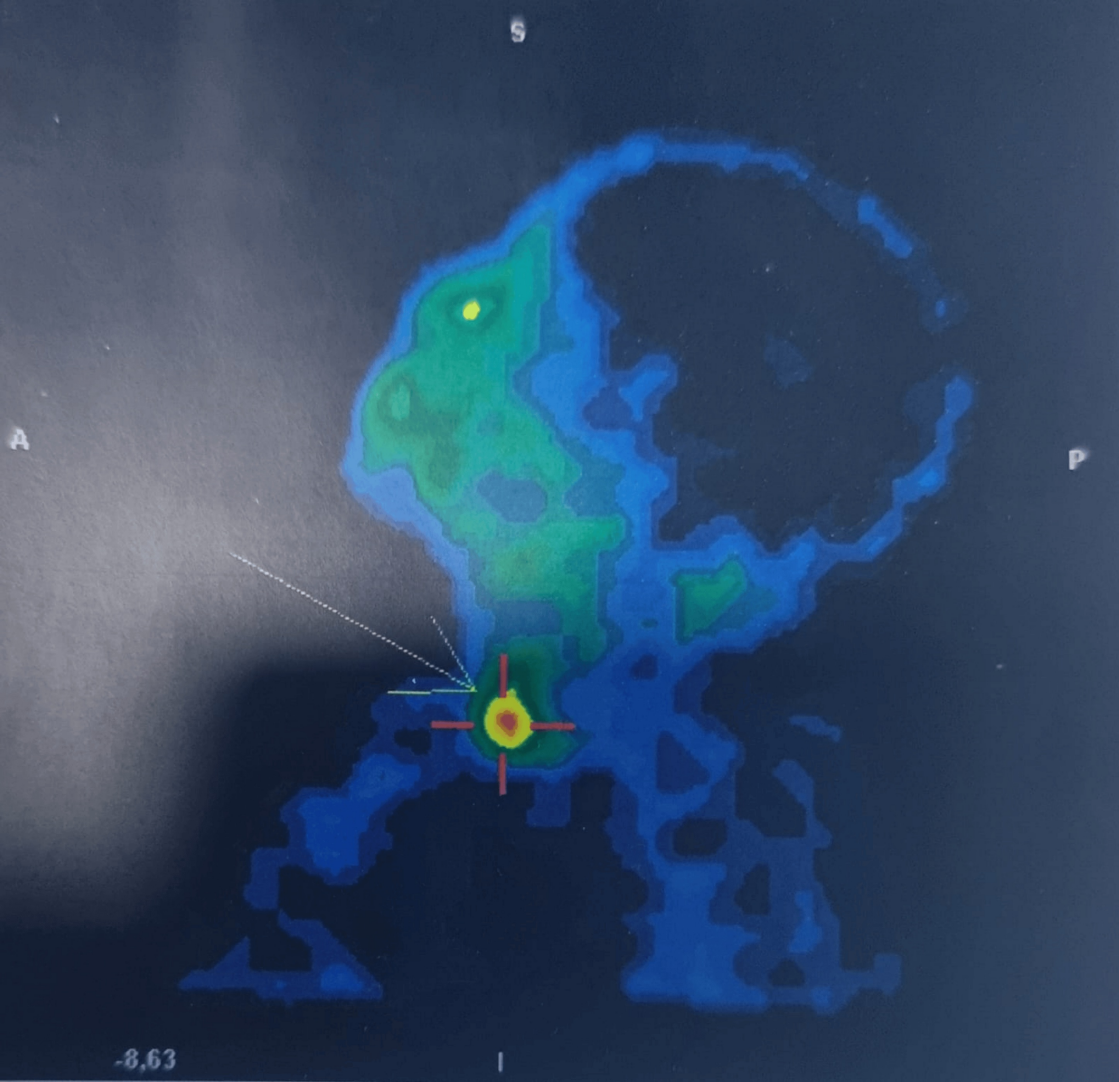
Parathyroid scintigraphy showing focal hyperfixation consistent with a left inferior parathyroid nodule (arrow).
